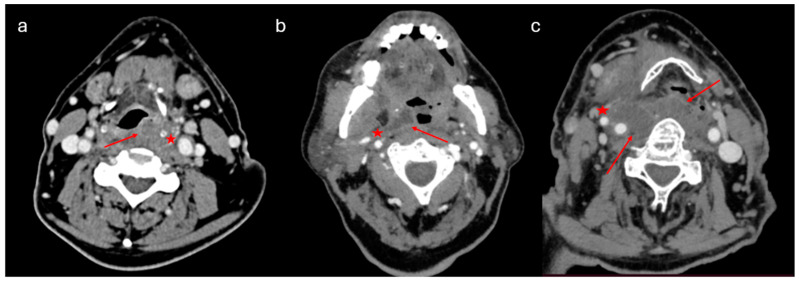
CT images of different categories of DNIs: (a) left-side PMA protruding into the pharyngeal lumen, passing medially to the hyoid bone; (b) right-side peritonsillar abscess, extending to the parapharyngeal space towards the right carotid artery and jugular vein; and (c) extended right-side parapharyngeal and retropharyngeal abscess. Arrows and stars indicate the margins of the abscess.
